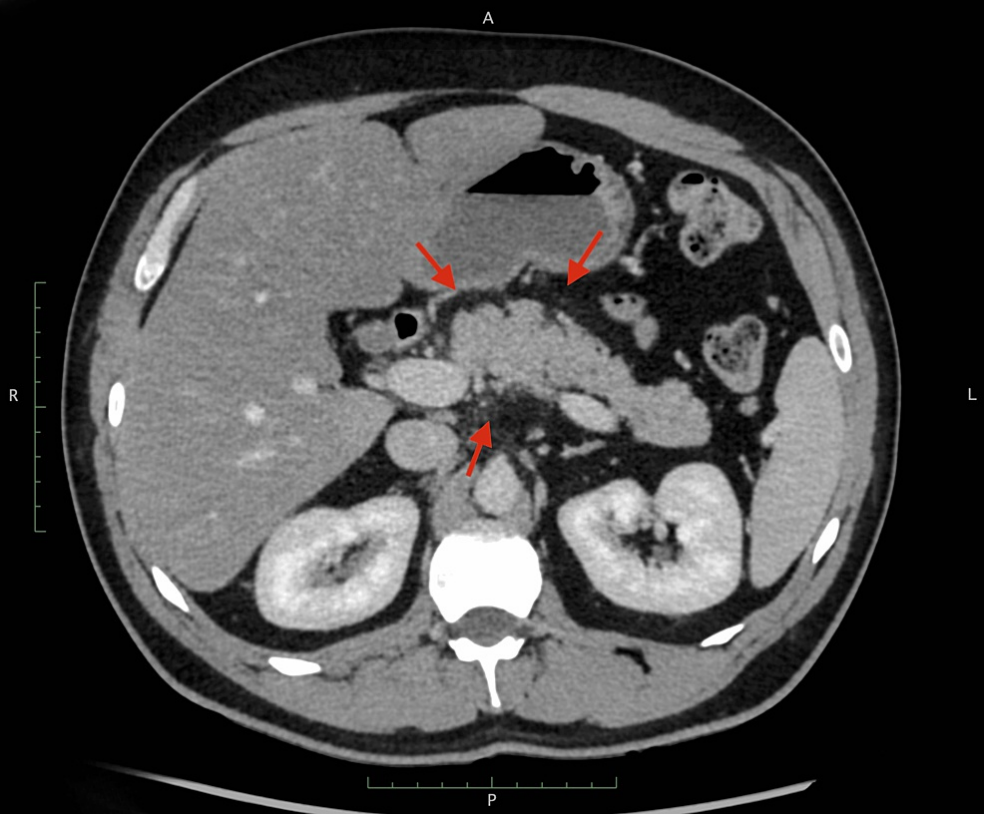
Contrast-enhanced CT scan of the abdomen showing subtle fat stranding around the pancreas (red arrows) consistent with pancreatitis CT: computed tomography
